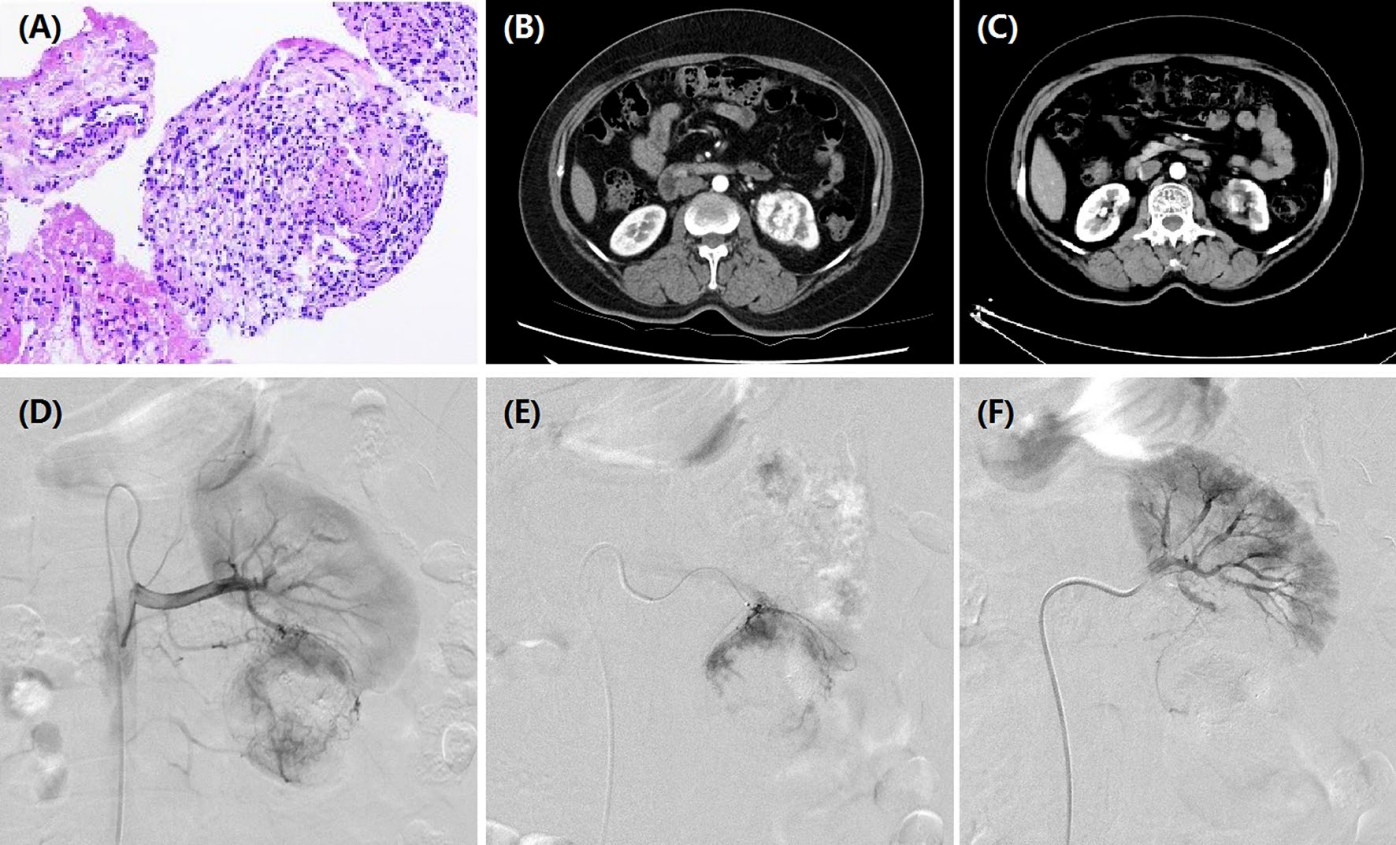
A 55-year female with left RCC treated by CB. (A) Pathological diagnosis of clear-cell type RCC in left kidney. (B) CT examination before operation revealed RCC of left kidney. (C) The left renal tumor was found to shrink after 3 months' follow-up. (D) A RCC was shown in left kidney by renal artery angiography. (E) The tumor-feeding artery was superselectively incubated via microcatheter. (F) The blood supply artery was embolized by the drug-loaded microspheres.
